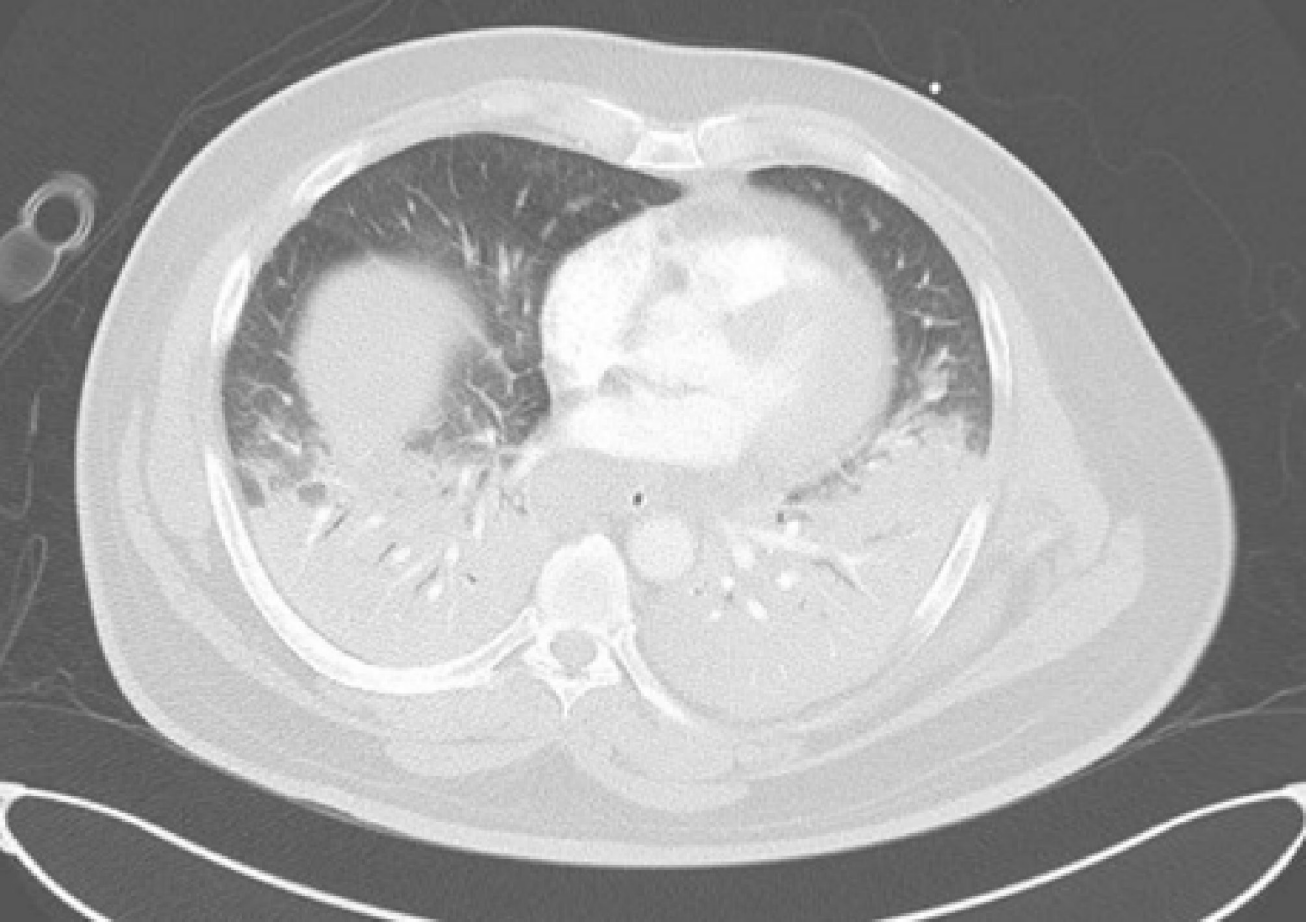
CT chest showing bilateral diffuse consolidation without pleural effusion.
